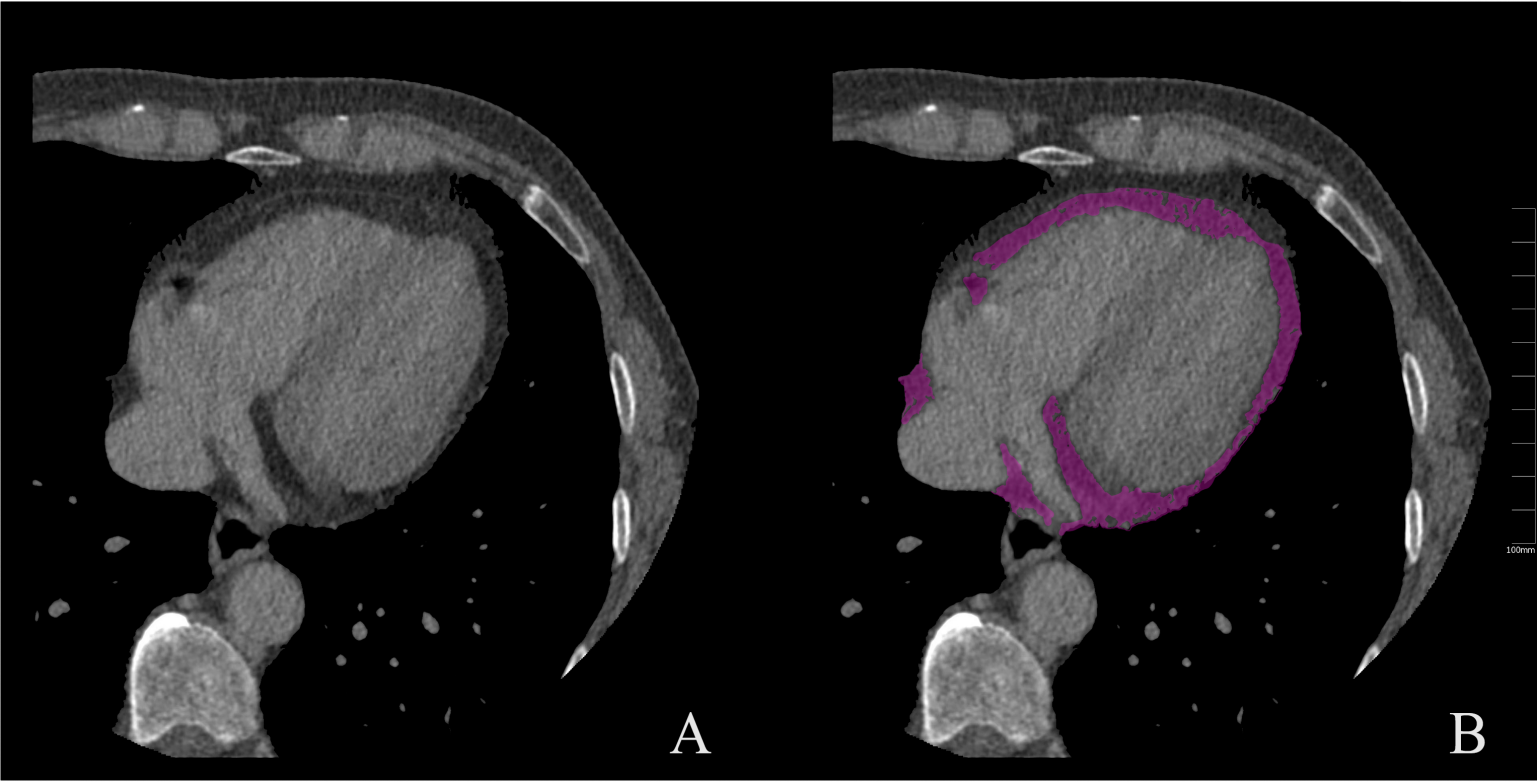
Measurement of EAT on CT images. (A) unenhanced CT image; (B)
